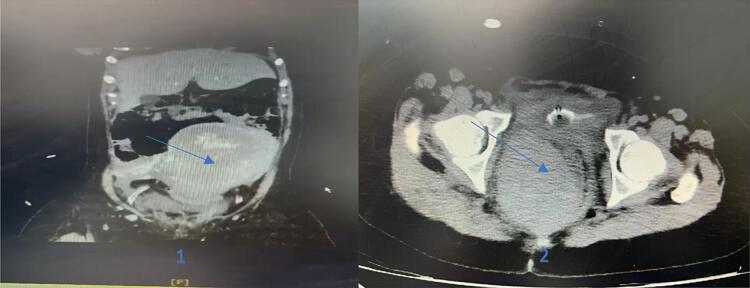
The abdomino-pelvic computed tomography (CT) scan of a large hematoma. 1) The coronal view, 2) the axial view, at right perirectal and presacral spaces extending upward to the pelvic inlet and lower pararenal space. The uterus and rectum were displaced to the left and anterior.
